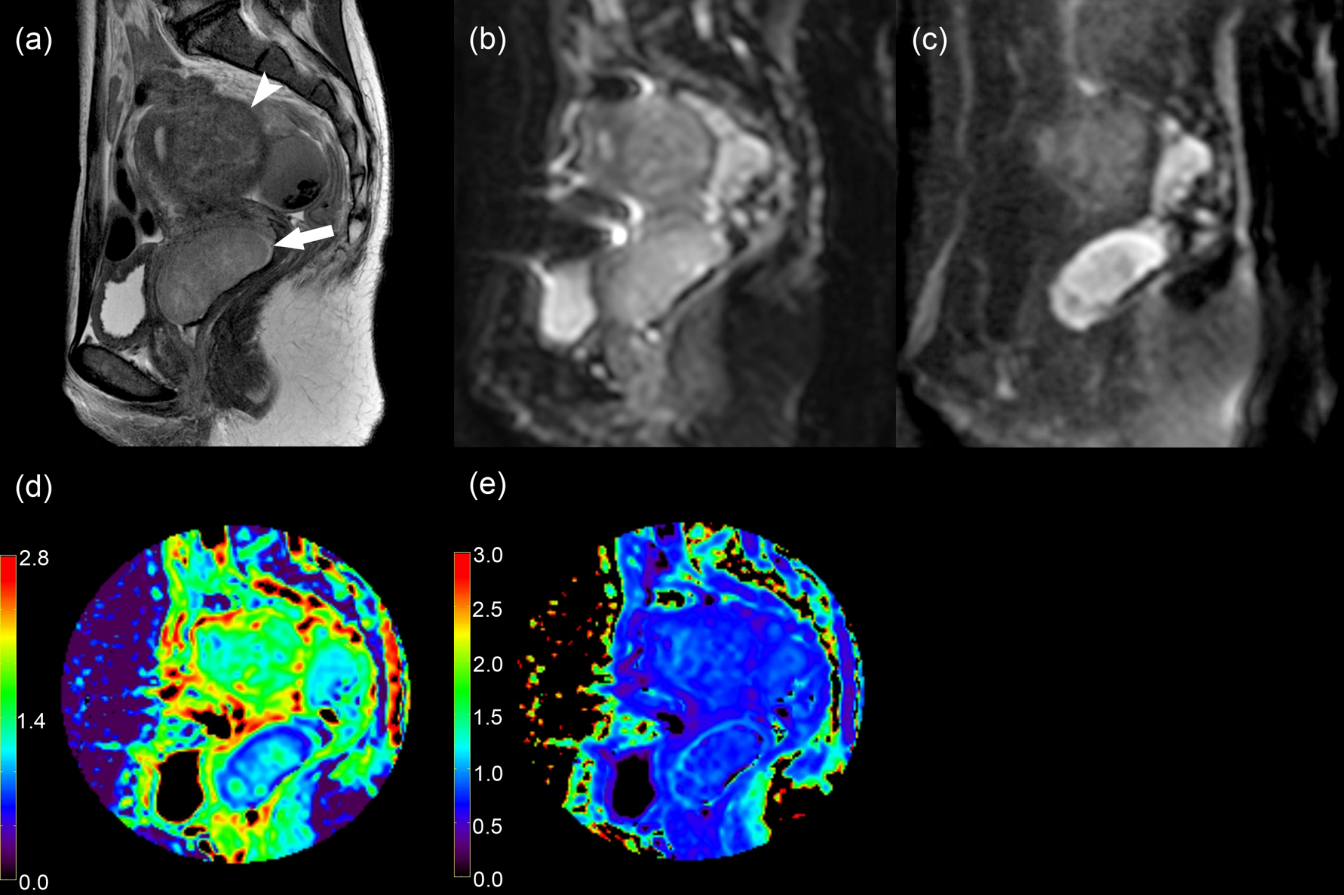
A 38-year-old woman with poorly differentiated carcinoma of the uterine cervix and coexisting posterior corporeal leiomyoma. (a) Sagittal T2-weighted image (TR/TE = 4500/80 ms), (b) sagittal diffusion-weighted image at *b* = 0 s/mm^2^, (c) sagittal diffusion-weighted image at *b* = 2500 s/mm^2^, (d) sagittal diffusivity map (D map), and (e) kurtosis map (K map) show the uterine cervical cancer (arrow). The tumor appears as a hyperintense mass on the diffusion-weighted image at *b* = 2500 s/mm^2^ with decreases in values on the D and K maps, whereas the leiomyoma (arrow head) has a low signal on the diffusion-weighted image, and an intermediate value on the D and K maps.
